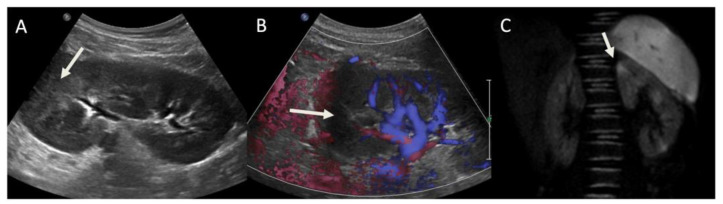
(A) US with color Doppler shows an area of hyperechoic parenchyma in the superior pole (arrow); (B) in the same area, a reduction of vascularization of color Doppler can be appreciated (arrow). (C) DWI shows multiple areas of restricted diffusion, in particular in the superior pole, where US alterations were detected (arrow).
